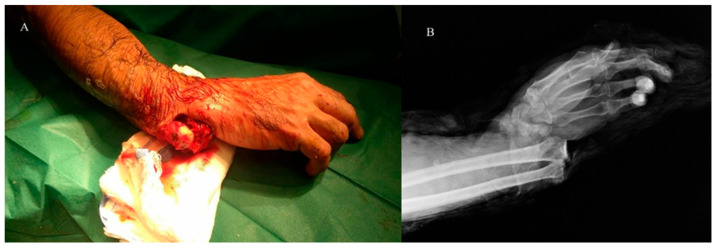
(A): The open injury upon presentation. (B): Anteroposterior X-ray view of the wrist. Dorsal radiocarpal dislocation of the right wrist (pre-reduction).
